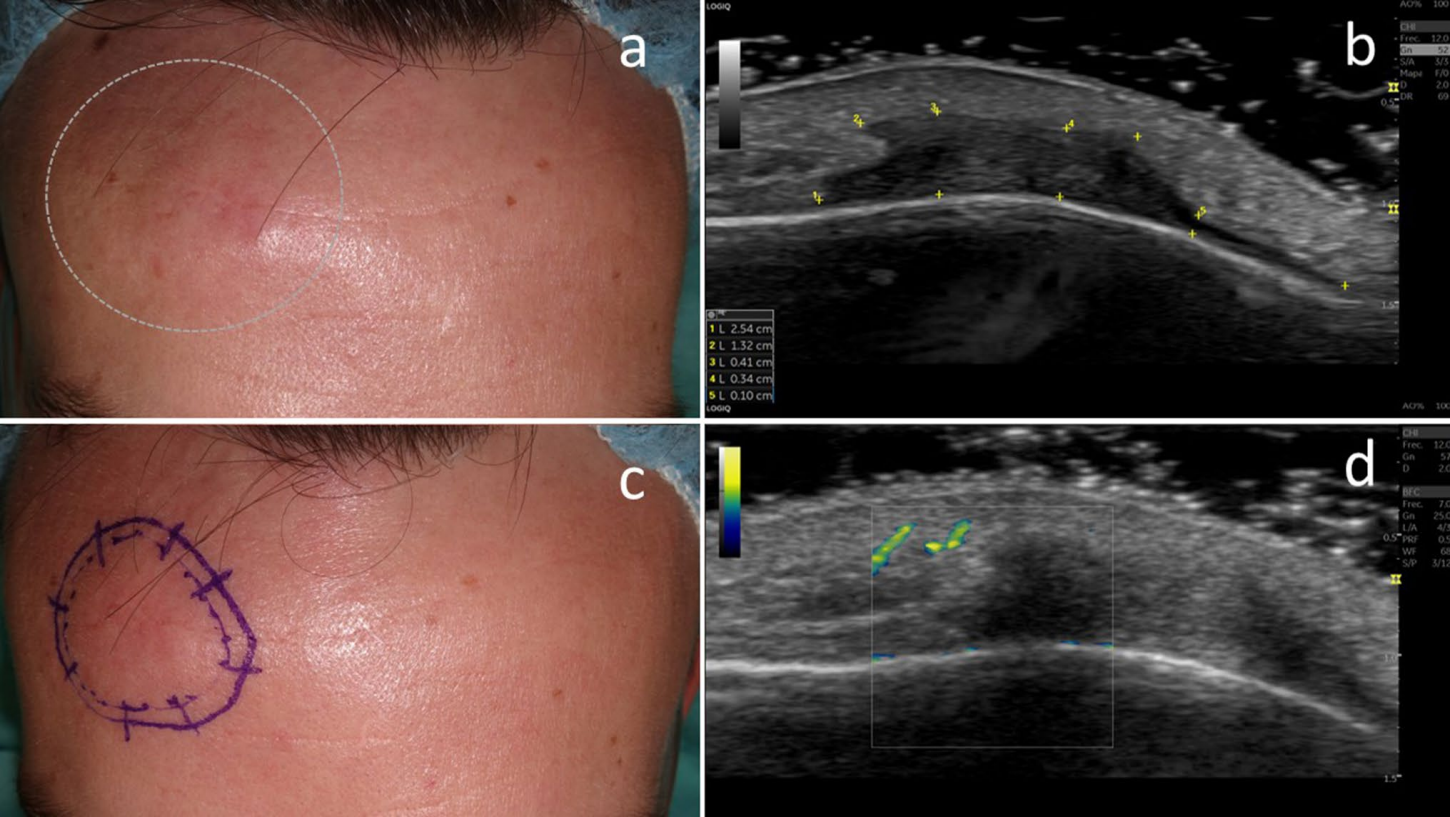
a Clinical picture of ill-defined subcutaneous tumour, in the right supraciliary area. b Transverse sonographic image showing a poorly defined asymmetric and hypoechoic mass located in dermis-hypodermis, with longest diameters of 2.5 × 0.4 cm. c Margins demarcation after ultrasound examination before starting the MMS surgery. Notice that the demarcated area extends beyond what was clinically suspected to the naked eye. d Color B-Flow Mode revealed slight increased vascularization exclusively at the periphery of the lesion
